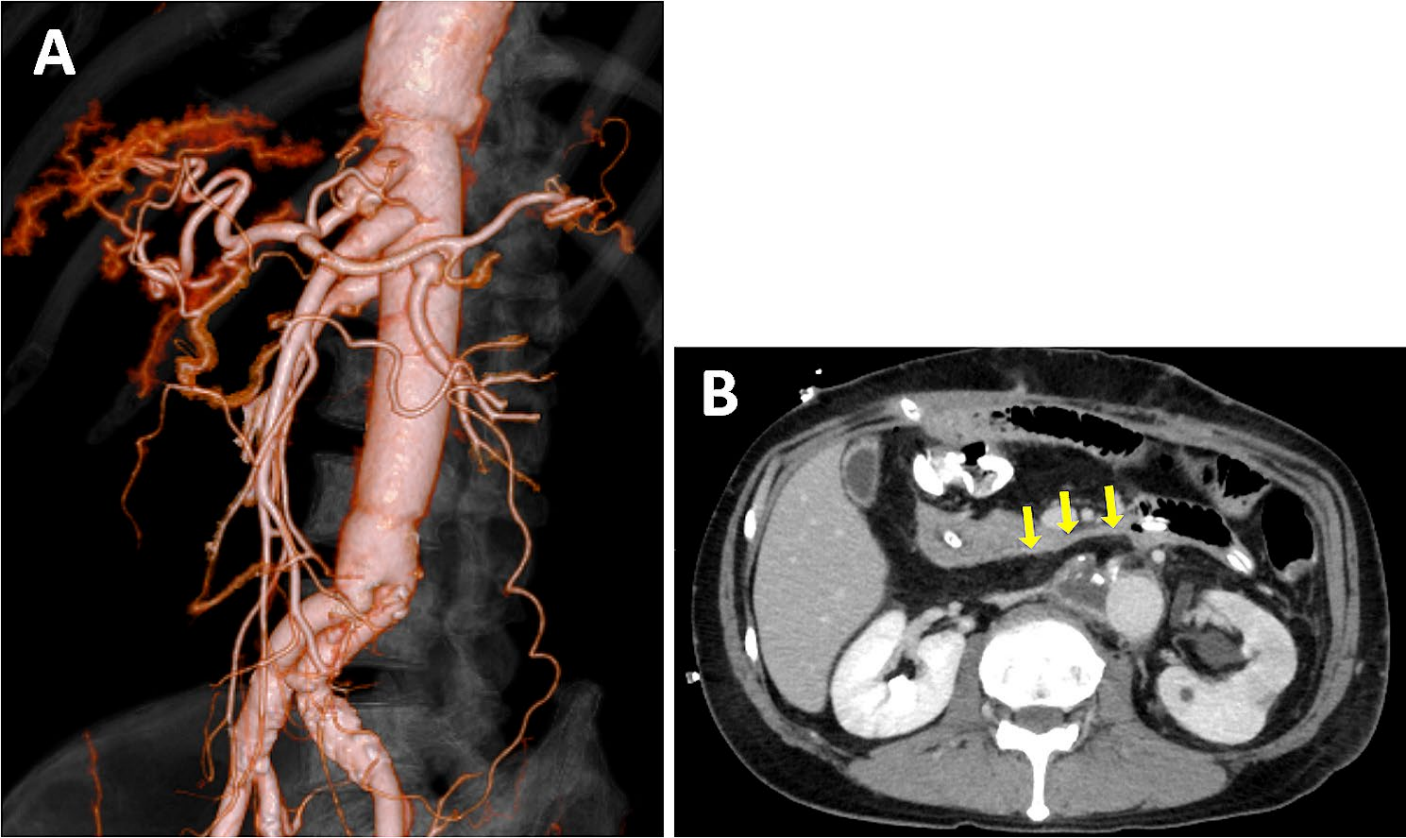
Postoperative computed tomography. A Three-dimensional view showing no graft occlusion. B Axial view demonstrating the omental flap between the graft and the duodenum(yellow arrow)
